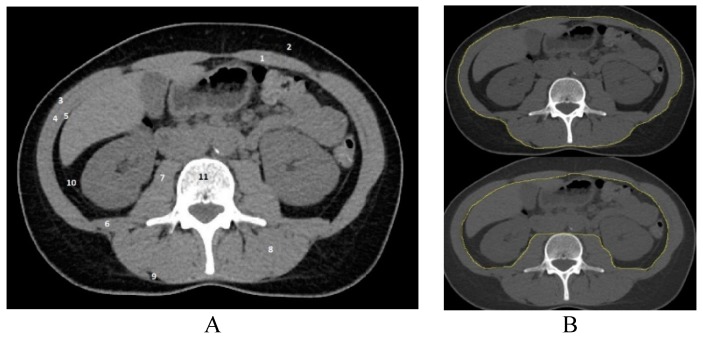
Axial CT image at the level of the L3 vertebra (A). Structures that are segmented for the assessment of sarcopenia: 1—m. rectus abdominis, 2—subcutaneous adipose tissue (SAT), 3—m. obliquus externus abdominis, 4—m. obliquus externus abdominis, 5—m. obliquus internus abdominis, 6—m. quadratus lumborum, 7—m. psoas major, 8—m. erector spinae, 9—intramuscular adipose tissue (IAT), 10—visceral adipose tissue (VAT), and 11—body of L3 vertebra. Axial CT image at the level of the L3 vertebra delineated with Image J “freehands selection” tool outer and inner abdominal musculature (B).
